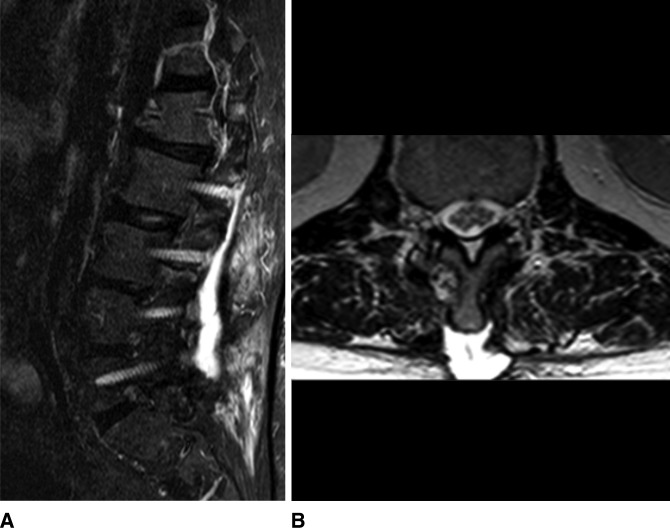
A, Sagittal STIR MRI lumbar spine. T2 hyperintense, peripherally enhancing fluid collection extending into pedicle screw tracts. B, Axial T2 MRI. Fluid collection in subcutaneous soft tissues extending to T12 spinous process.
